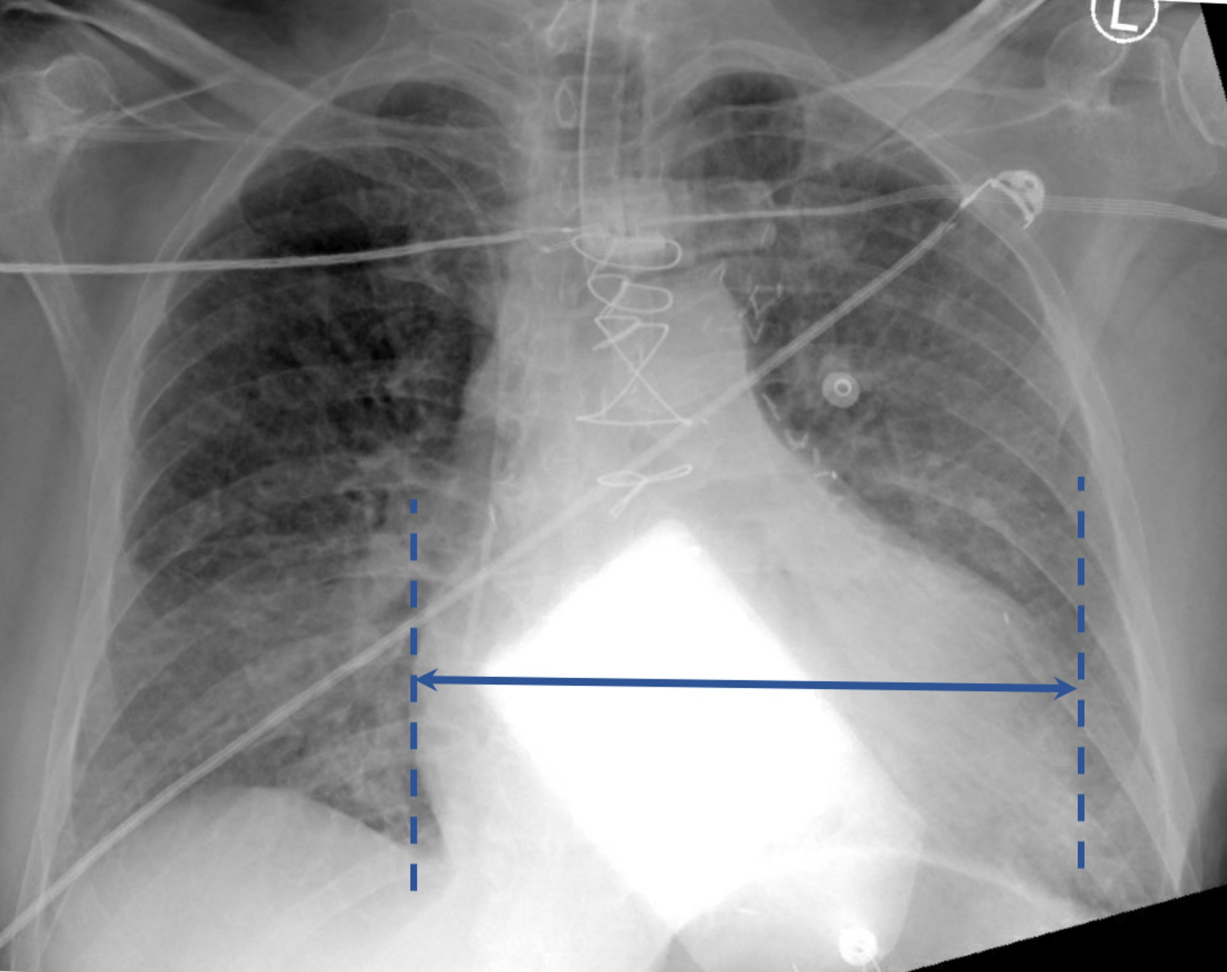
Portable chest x-ray demonstrating enlarged cardiac silhouette (arrow), pulmonary edema, and midline sternotomy wires.
